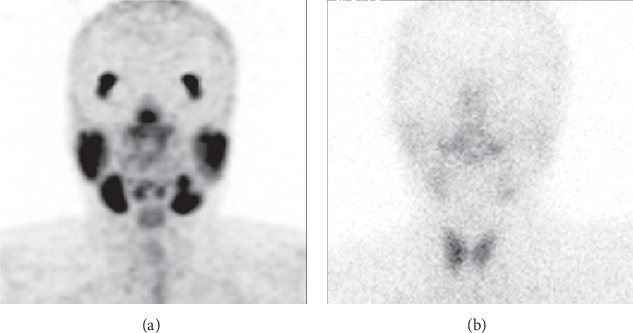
A 43-year-old female (patient 14) with nasopharyngeal carcinoma after radiotherapy and chemotherapy. ^68^Ga-PSMA-11 PET/CT MIP (a) shows that the imaging agents were evenly and symmetrically distributed in bilateral lacrimal glands and major salivary glands (right parotid gland, left parotid gland, right submandibular gland, and left submandibular gland; SUVmax values of 8.1, 8.0, 10.2, and, 8.2, respectively). No obvious abnormalities were observed. However, ^99m^TcO_4_^−^ SGS (b) shows a mild-to-moderate decrease in the uptake function of the left parotid (right parotid gland, left parotid gland, right submandibular gland, and left submandibular gland; UR values of 3.1, 2.2, 3.4, and 2.8, respectively).
